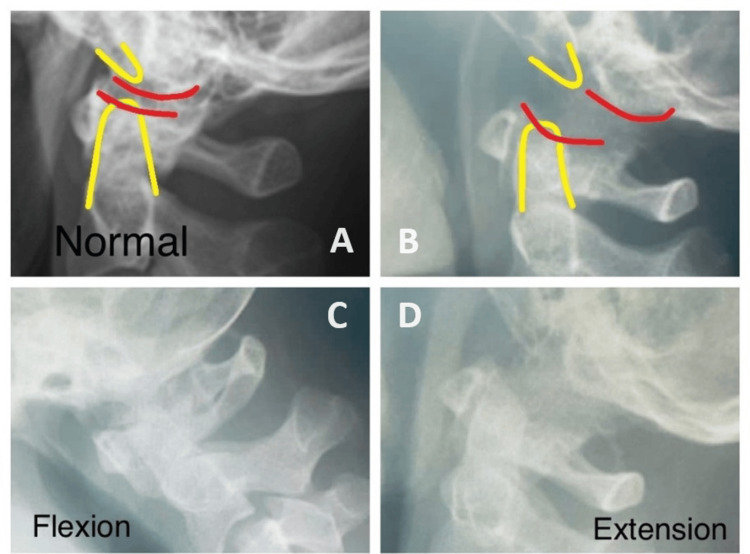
Comparison of lateral view cervical x-ray of the patient in neutral (B), flexion (C), and extension (D) with the ideally normal x-ray (A). Extension and neutral views show atlantooccipital subluxation (AOS). Flexion view shows partial reduction of AOS.
